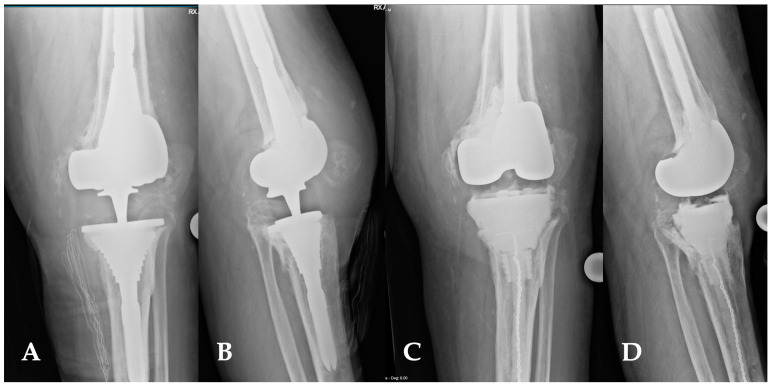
(A,D) This image depicts the comparison between preoperative and postoperative X-rays of a patient affected by periprosthetic joint infections of the knee (A,B). The patient’s characteristics met the inclusion criteria for the indication of a balanced articulating spacer. The spacer was constructed using cones and both asymmetrical and symmetrical augments, particularly to address the severe bone loss of the tibia (C,D).
